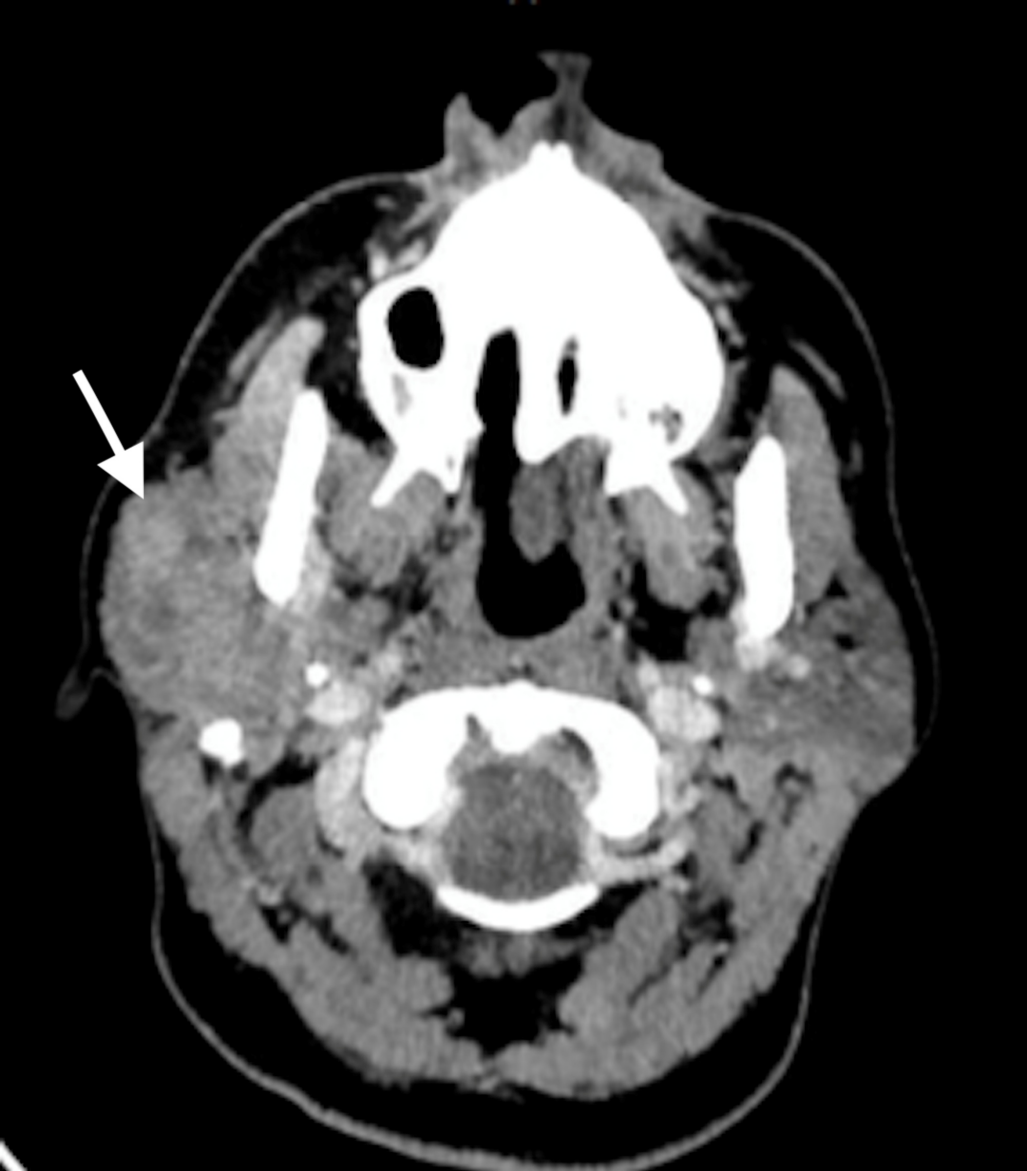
Contrasted CT neck image in axial view The image shows an infiltrating heterogeneously enhancing mass of the superficial and deep lobes of the right parotid gland with cervical lymphadenopathy of level 2 (arrow) CT: computed tomography
